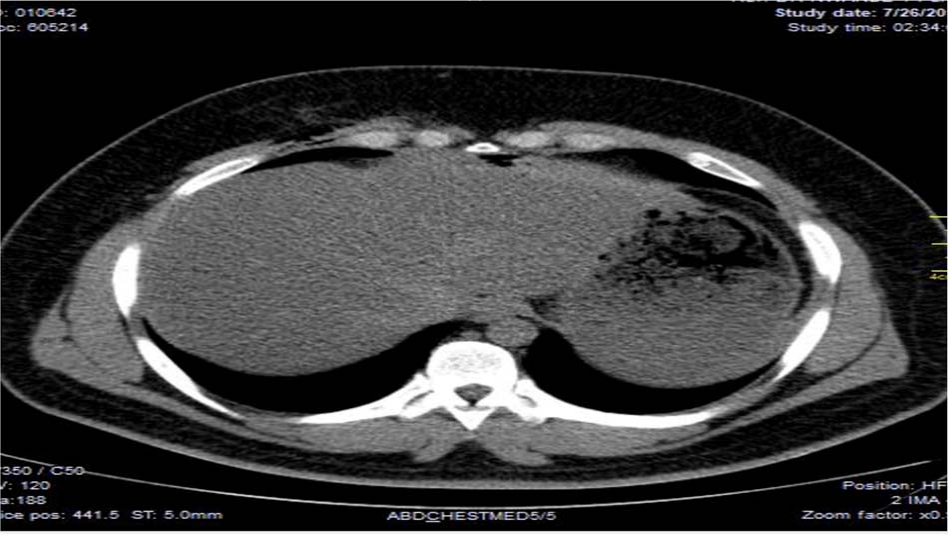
Image showing air lucencies in the right intercostal muscles and subcutaneous tissue around the lower right parasternal region.
